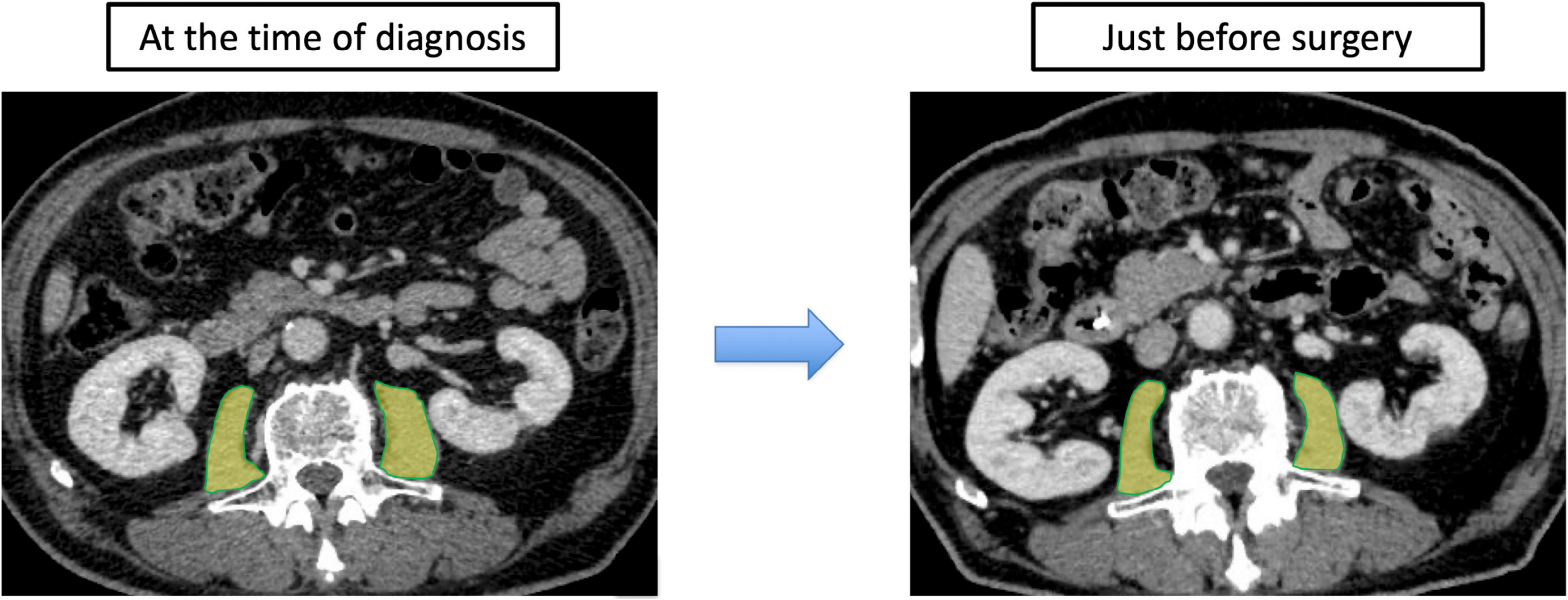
Measurement of the psoas muscle area (PMA) at the time of diagnosis and just before surgery. The bilateral psoas muscle cross‐sectional computed tomography images at the middle‐level of the third lumbar vertebra was measured using SYNAPSE VINCENT software. The border of the bilateral psoas muscle was manually outlined and quantified using Hounsfield unit thresholds (−29 to +150). The PMA just before surgery was divided by that at the time of diagnosis, and it was defined as the rate of change of PMA (CPMA)
